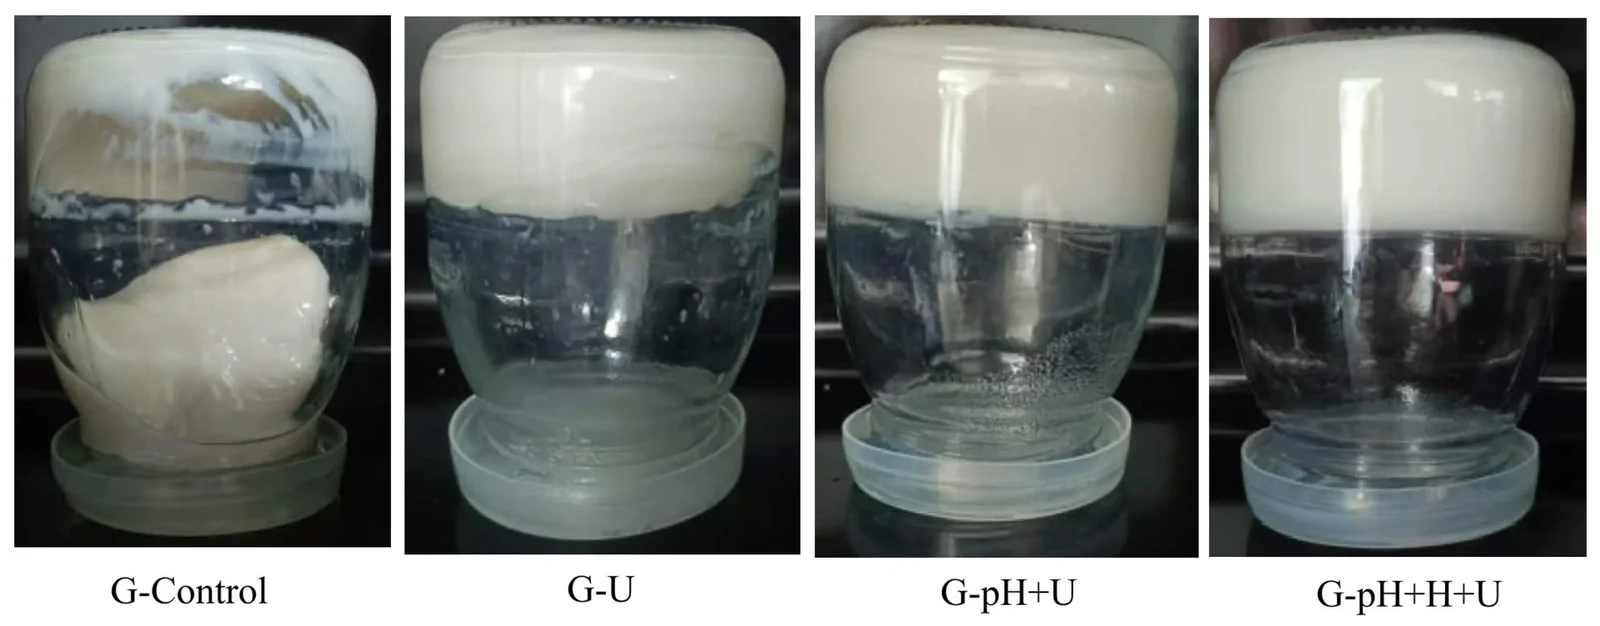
Photographs of perilla seed protein gels subjected to high-pressure homogenization, pH-shifting, and ultrasound treatments. The designations G-U, G-pH+U, and G-pH+H+U denote gels obtained from the respective modification conditions. Treatment labels are the same as in Figure 1.
